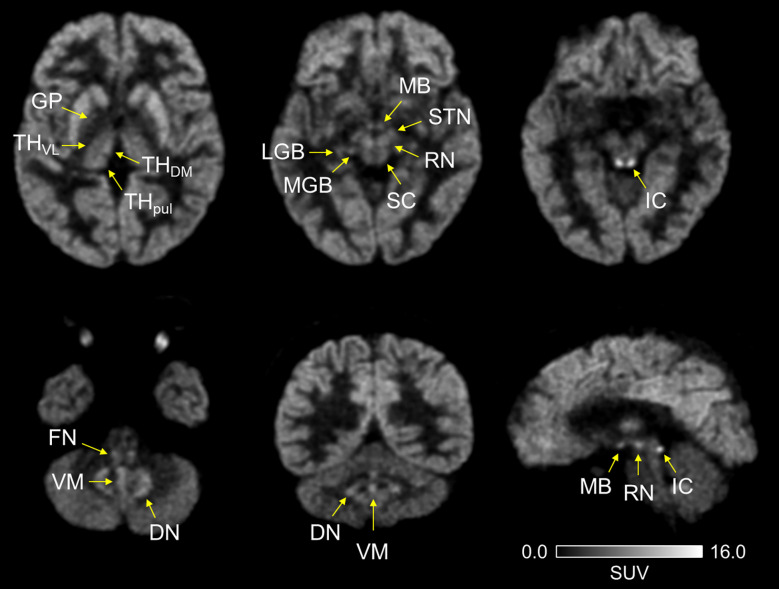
Brain [^18^F]FDG PET in cognitively normal volunteer, demonstrating high-quality images with well-defined cortical uptake and symmetric distribution of glucose metabolism in gray matter, deep cortical structures, and brainstem nuclei. Anatomic structures were visually identified by a nuclear medicine physician. DN = dentate nucleus; FN = fastigial nucleus; GP = globus pallidus; IC = inferior colliculus; LGB = lateral geniculate body; MB = mammillary body; MGB = medial geniculate body; RN = red nucleus; SC = superior colliculus; STN = subthalamic nucleus; TH_DM_ = dorsomedial thalamic nucleus; TH_pul_ = pulvinar nucleus; TH_VL_ = ventrolateral thalamic nucleus; VM = vermis.
